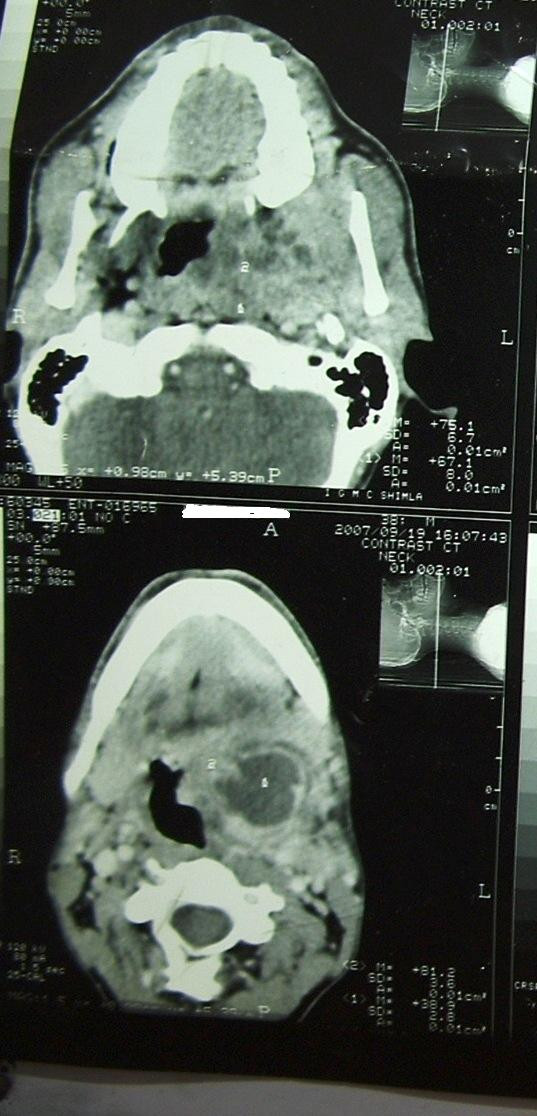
On 19^th ^Sep 2007, pre-radiotherapy contrast CT scan found heterogeneous enhanced tumor in the nasopharynx with extension up to parapharyngeal area. The tumor had multiple necrotic areas with largest area in its lower part.
